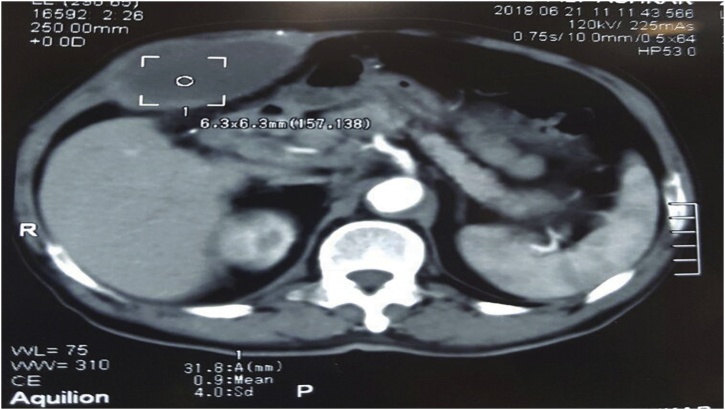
CT scan showing the cystic mass in the abdominal wall.
